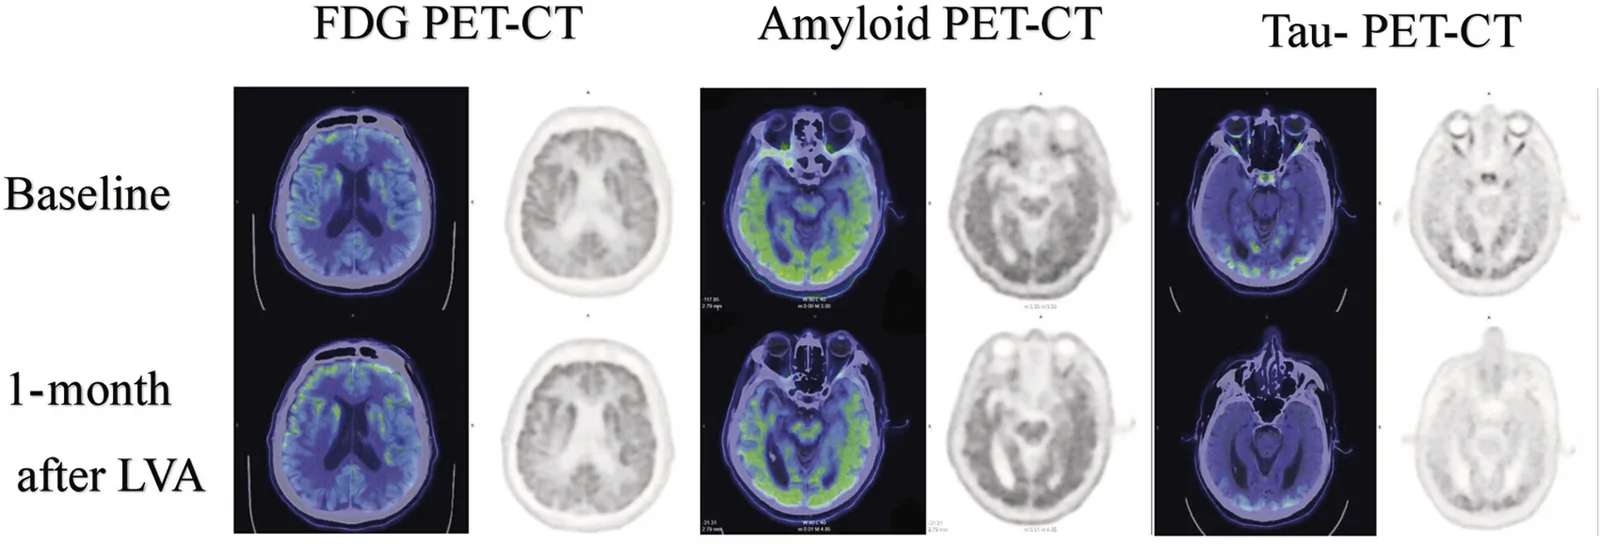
Trends of SUVr values across 19 brain regions on FDG-PET scans at baseline and postoperatively. FDG, fluorodeoxyglucose; PET, positron emission tomography; SUVr, standardized uptake value ratio.
